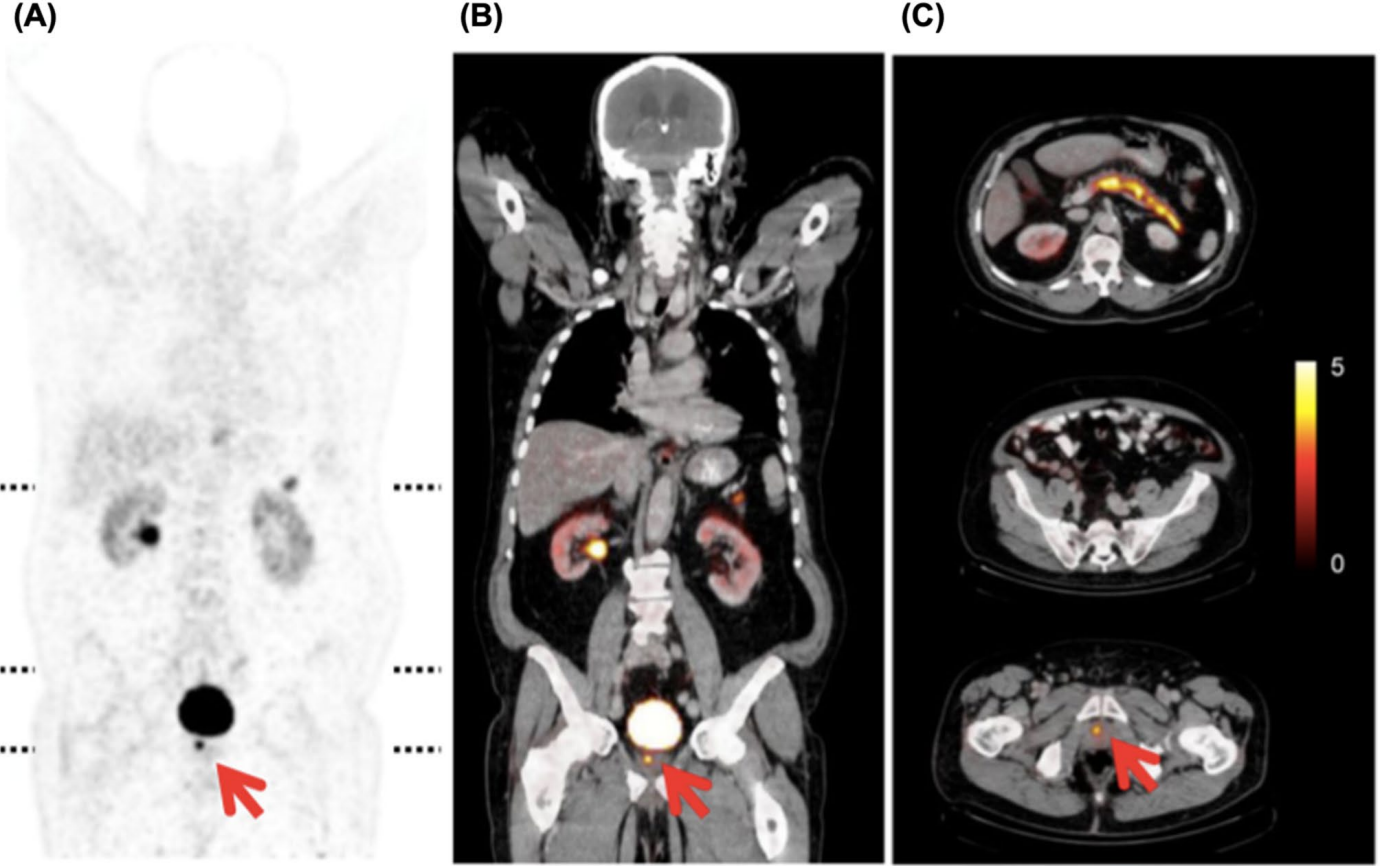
[^64^Cu]Cu-CB-TE2A-AR06 PET/CT images acquired 4 h post-injection. Coronal PET (A) and fused PET/CT (B) sections demonstrate intense tracer uptake in the prostate tumor (red arrows) and physiological uptake in the pancreas. Axial PET/CT fusion images (C) correspond to the levels indicated by the dotted lines in (A). Only low uptake is seen in the kidneys, liver, and intestines. PET images are scaled to a maximum SUV of 5 (color bar). Reproduced from Wieser et al. [148], licensed under CC BY-NC-ND 3.0 (https://creativecommons.org/licenses/by-nc-nd/3.0/)
